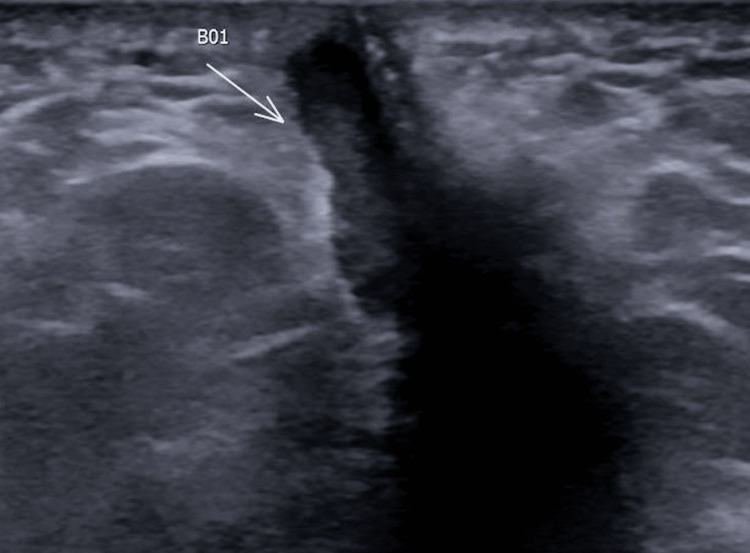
Pelvic ultrasound showing a hypoechoic, hypervascular area to the left of midline perineum (arrow) extending to the anus, measuring approximately 4.6 x 0.9 x 1.0 cm
